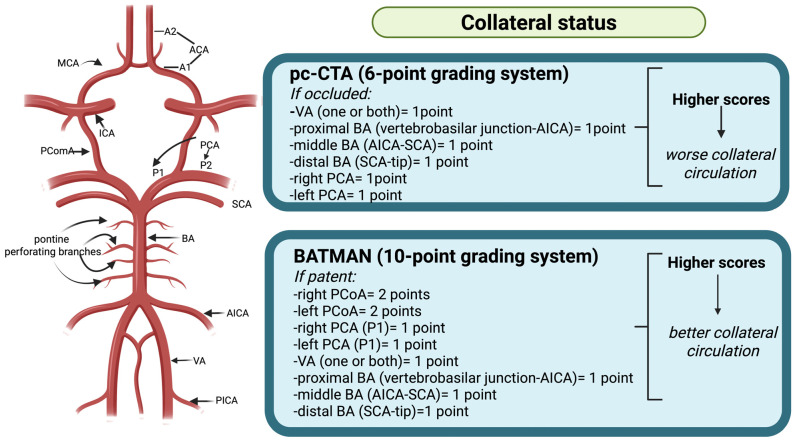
Evaluation of collateral status on computed tomography angiography (CTA) using the Posterior Circulation CTA (pc-CTA) score and basilar artery (BATMAN) score.
